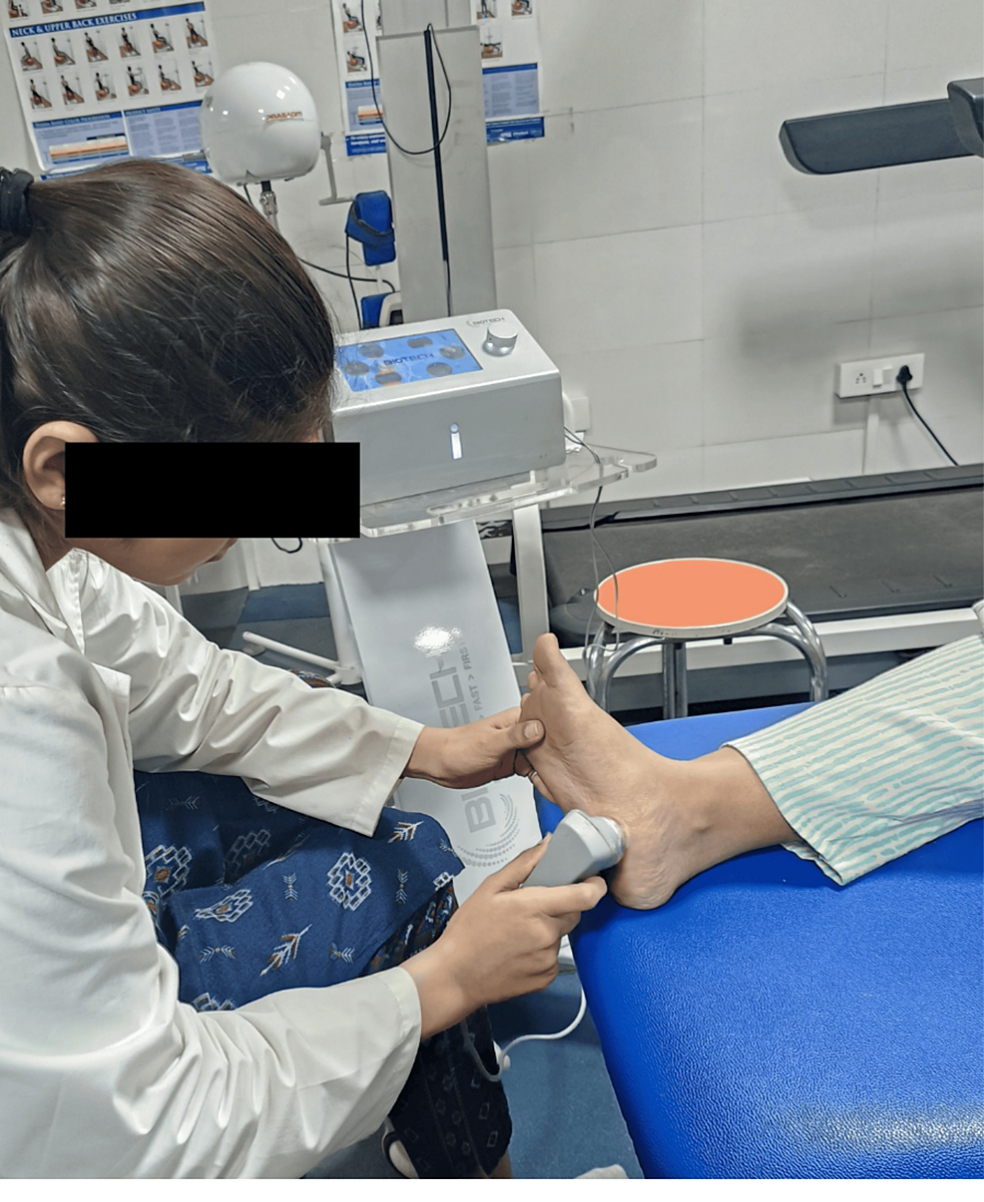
The treatment of plantar fasciitis pain using ultrasound with a frequency of 1 megahertz and therapeutic intensity of 1.8 watts per square centimetre.
